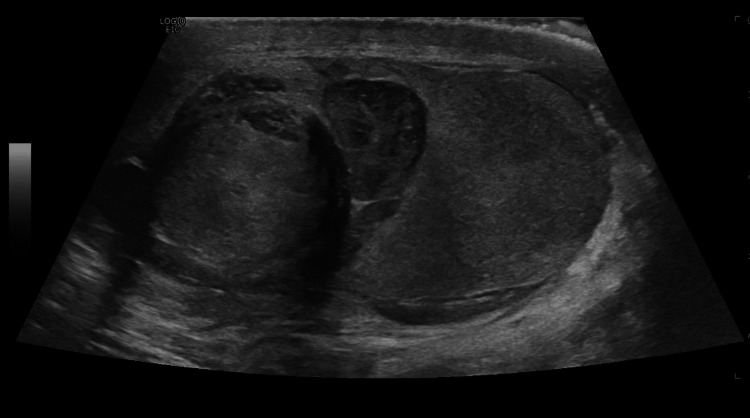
Ultrasound of the testis demonstrating rupture of testis with associated hematoma
